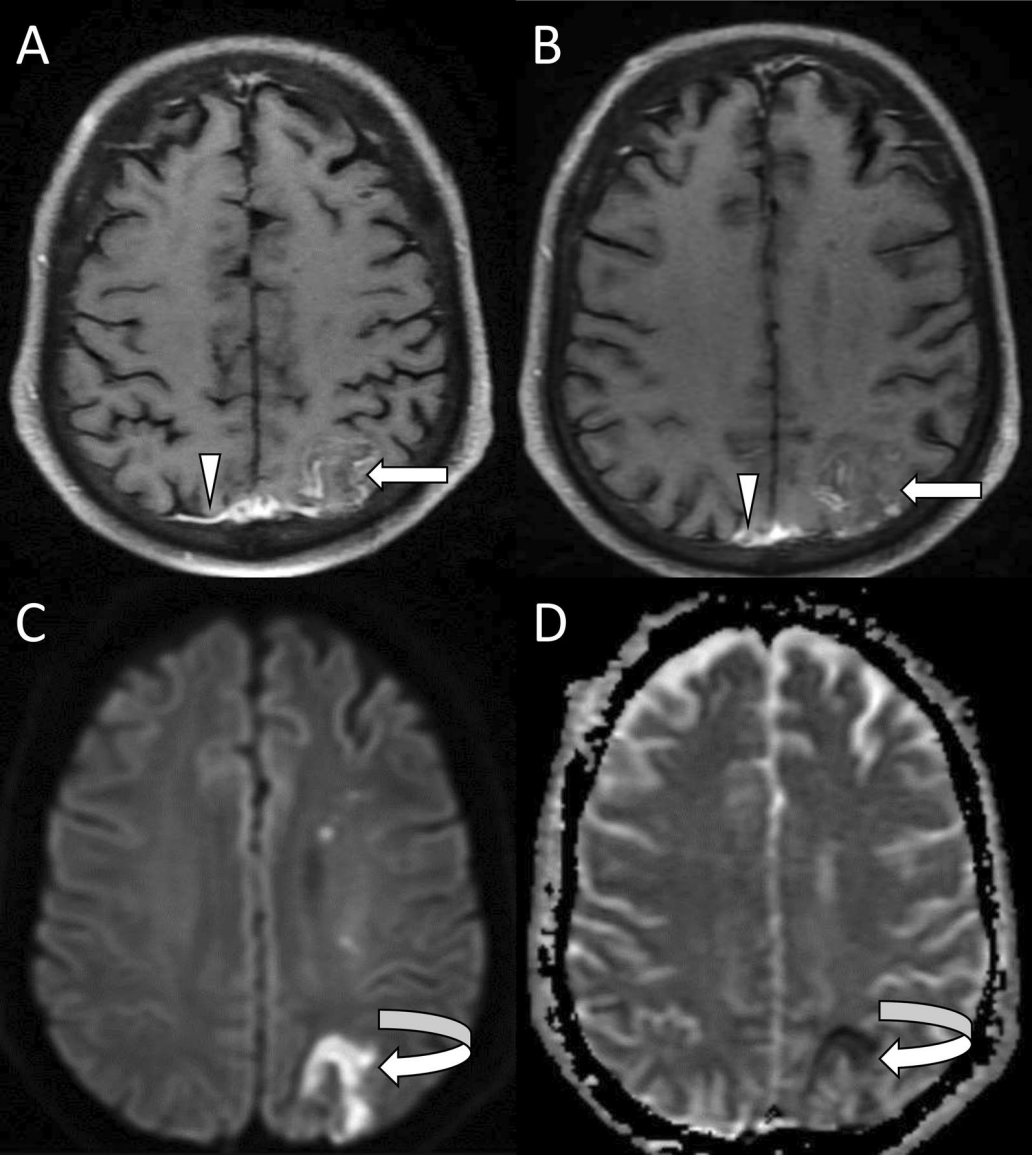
Rhinocerebral mucormycosis with late cerebritis. On contrast-enhanced axial T1-weighted fat-saturated images (A, B), the lesion shows mild incomplete peripheral enhancement (straight white arrows) with enhancement of the adjacent dura (arrowhead). Diffusion-weighted and apparent diffusion coefficient (ADC) images (C, D) show peripheral areas of restricted diffusion (curved white arrows)
